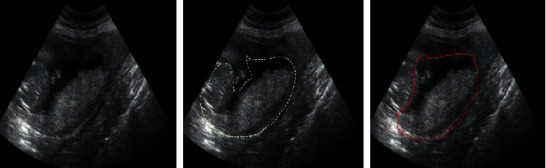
Ultrasonic positioning of placenta previa based on the intelligent recognition algorithm.
